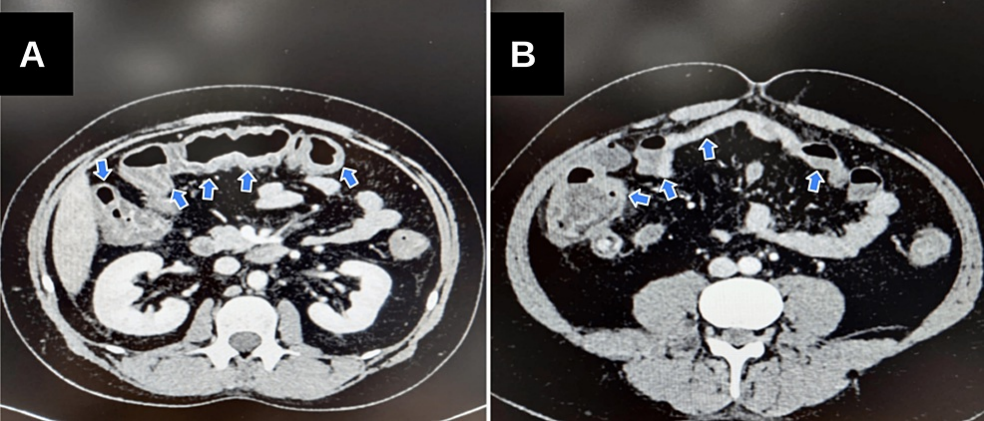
Transverse section of a contrast CT abdomen showing pancolitis with inflammatory stranding of pericolic mesentery in left colon and mucosal oedema in right colon (arrows)
